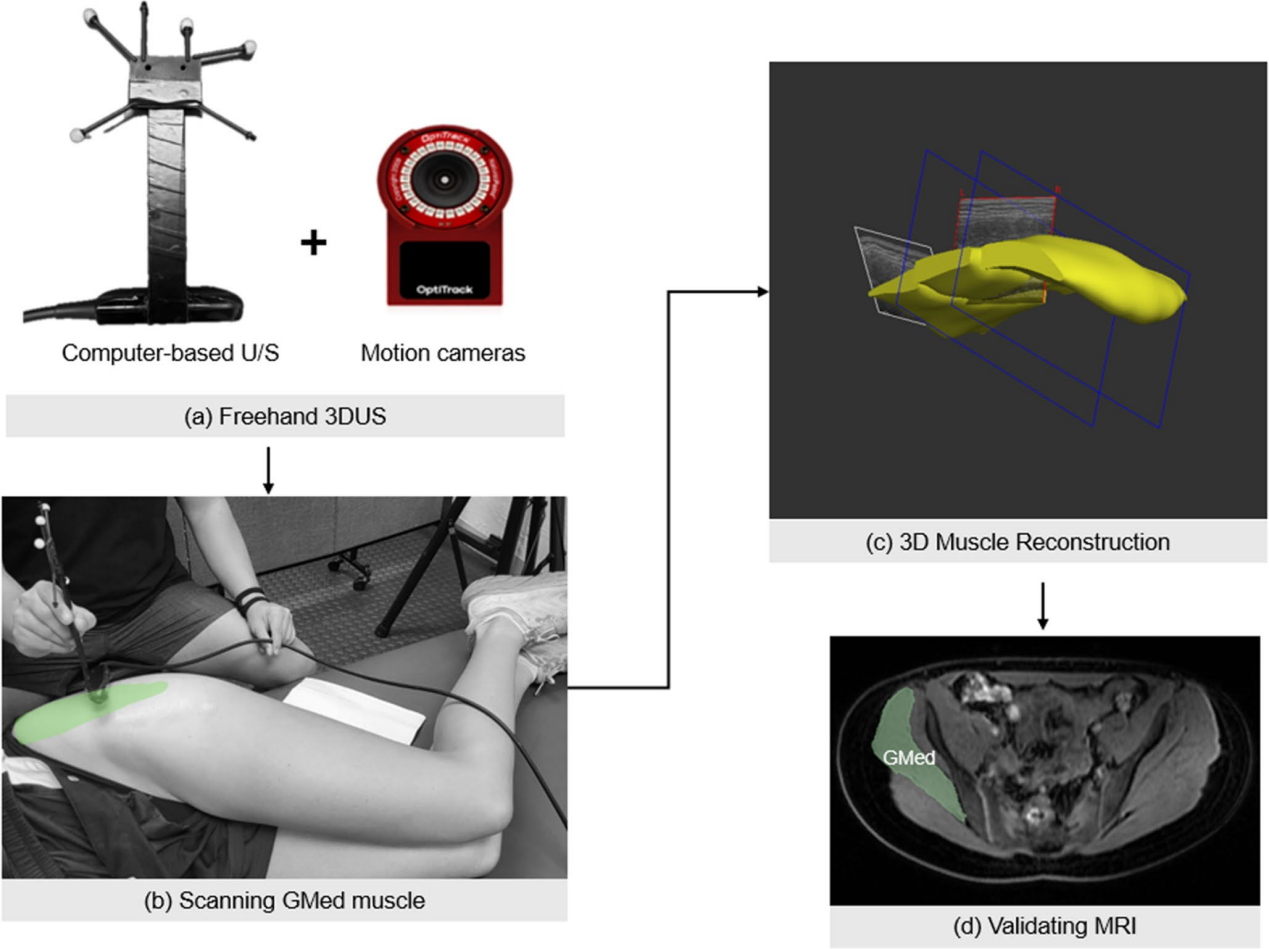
(a) Ultrasound device and motion capture cameras for tracking transducer position (b) The GMed muscle was scanned using a tracked ultrasound probe (c) 3D muscle reconstruction (d) A representative axial MRI slice with the GMed segmented in green
